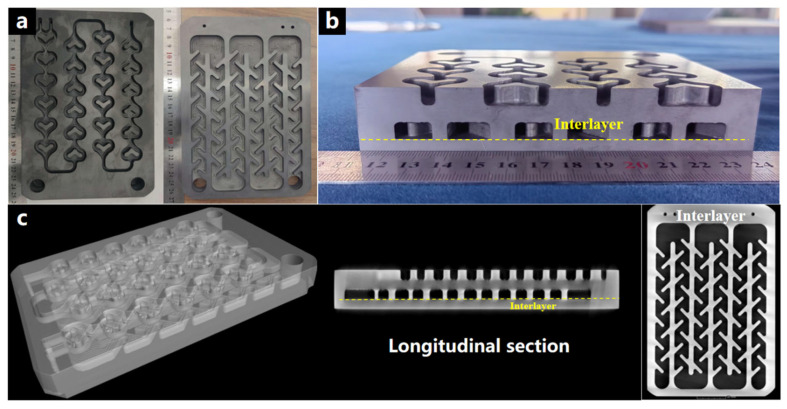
(a) Photos of SiC ceramic parts for joining (b) Sample profile photo after joining and (c) Industrial CT nondestructive testing.
